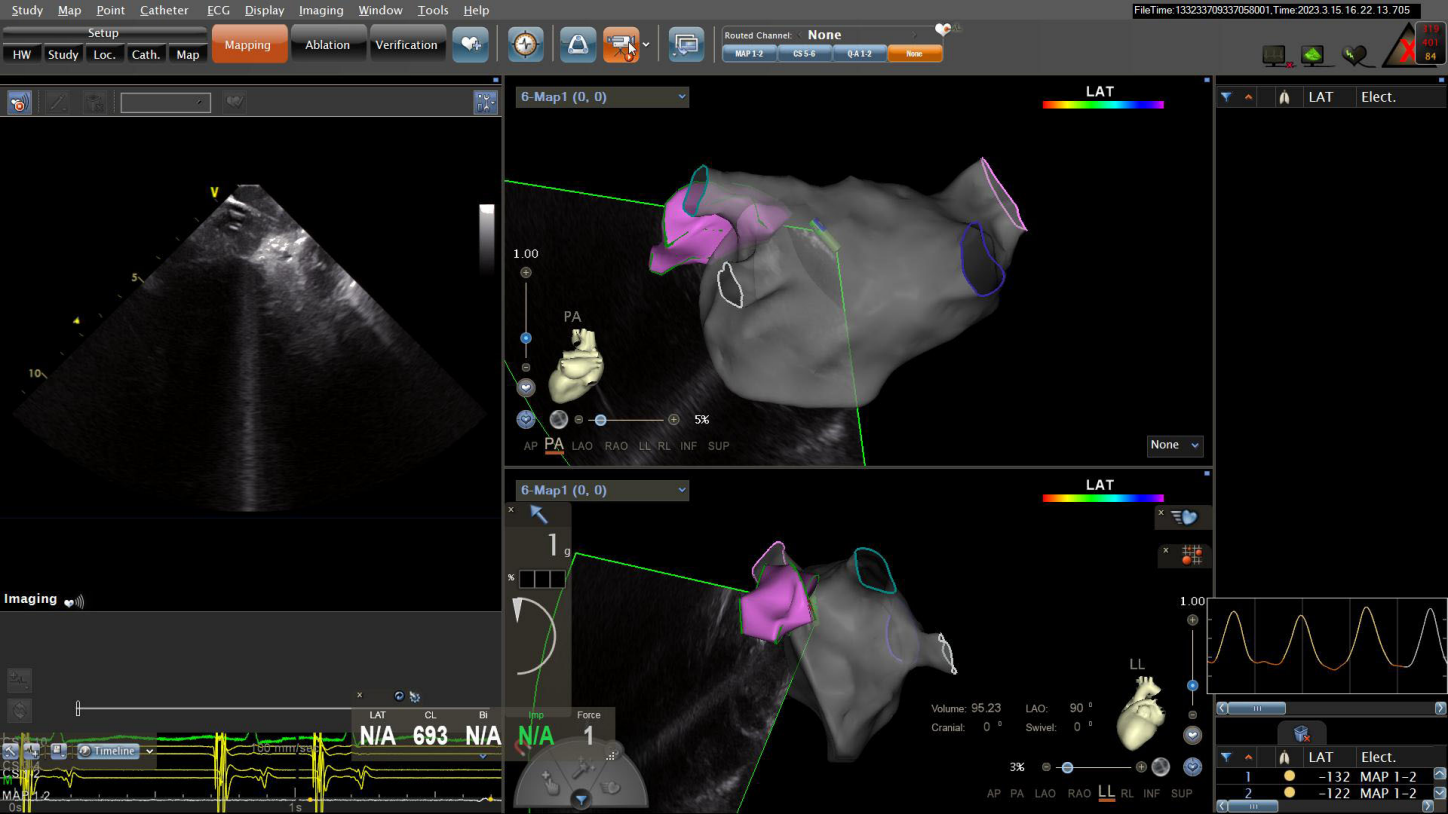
Utilizing intracardiac echocardiography to model the left atrial
